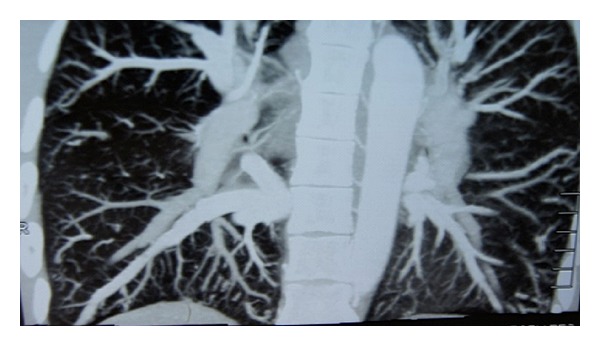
Pulmonary computed tomographic angiography (CTA) shows pulmonary arteriovenous malformation.
